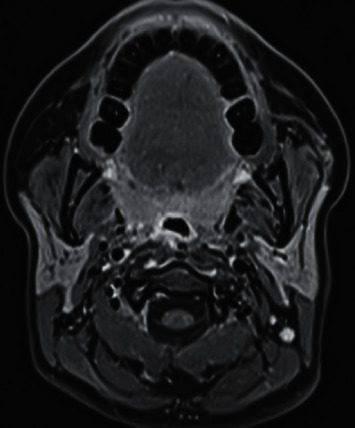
Postoperative (18-months) magnetic resonance imaging axial T1 postcontrast with fat suppression showing complete excision of right parapharyngeal cyst with no evidence of recurrence.
